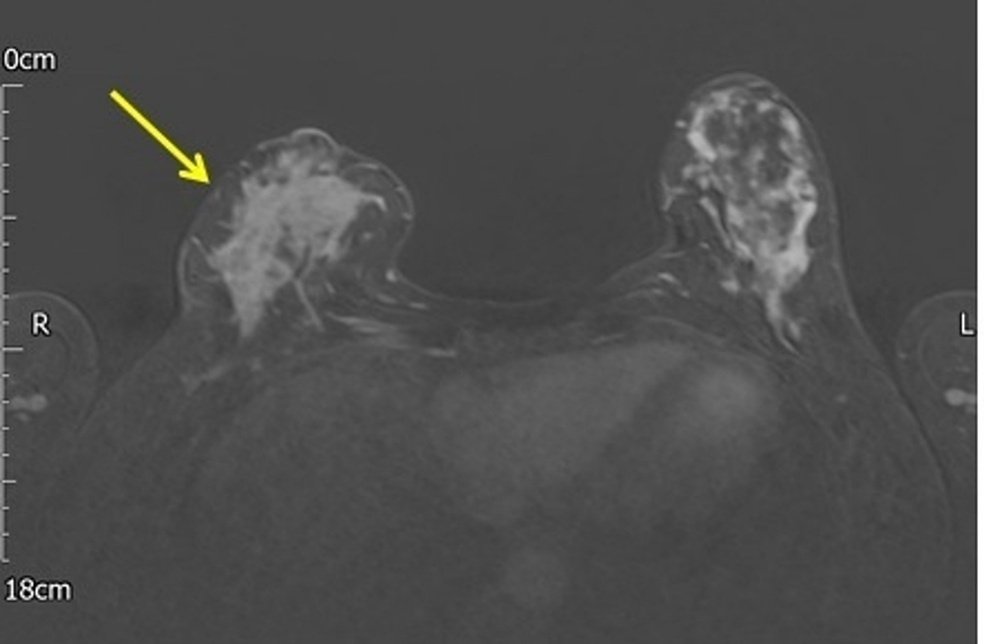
MRI of the breast showing large areas of enhancement involving the entire right breast (yellow arrow). MRI: magnetic resonance imaging
